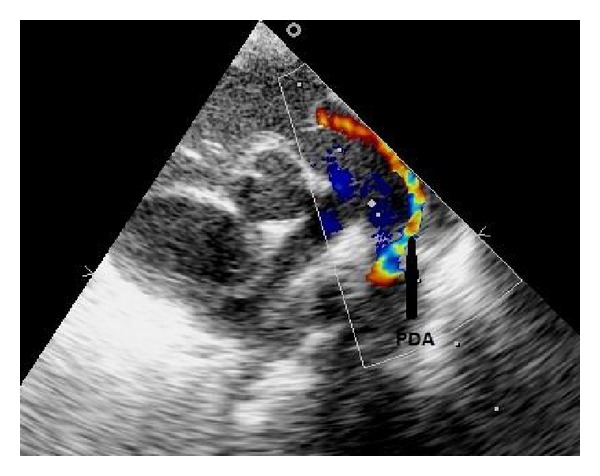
Transthoracic echocardiogram, parasternal short axis view showing patent ductus arteriosus.
